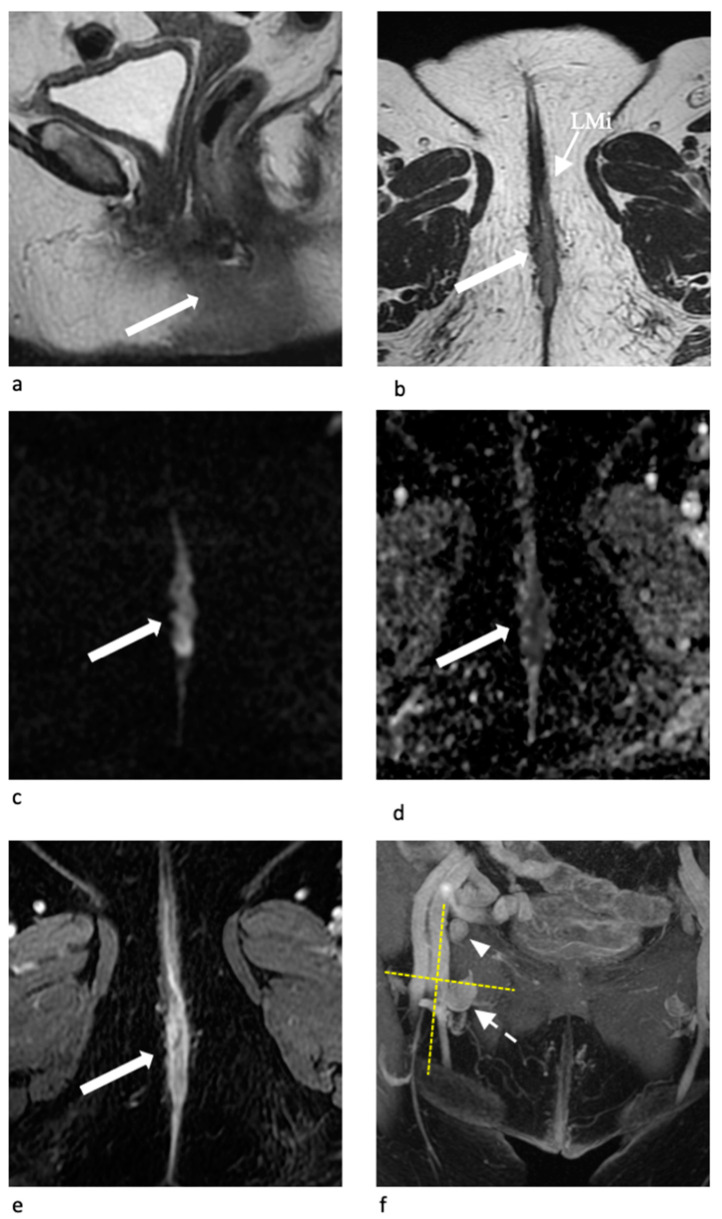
Stage III, in a 72-year-old woman with vulvar carcinoma. Sagittal (a) and axial T2-W (b) MR images show a midline vulvar mass (arrow) involving labia minora (LMi) and confined in the perineum without invasion of adjacent organs. Axial DW-MR image (c) and corresponding ADC map (d) show hyperintensity of the lesion with marked diffusion restriction. Axial contrast-enhanced T1-W MR images (e) show the early arterial enhancement of the tumor. On coronal maximum intensity projection (MIP) reformatted MR image (f) there are an enlarged right inguinal node in the IV Daseler level (dotted arrow) and a smaller one in the I level (arrowheads). Surgery was performed and stage III was confirmed.
